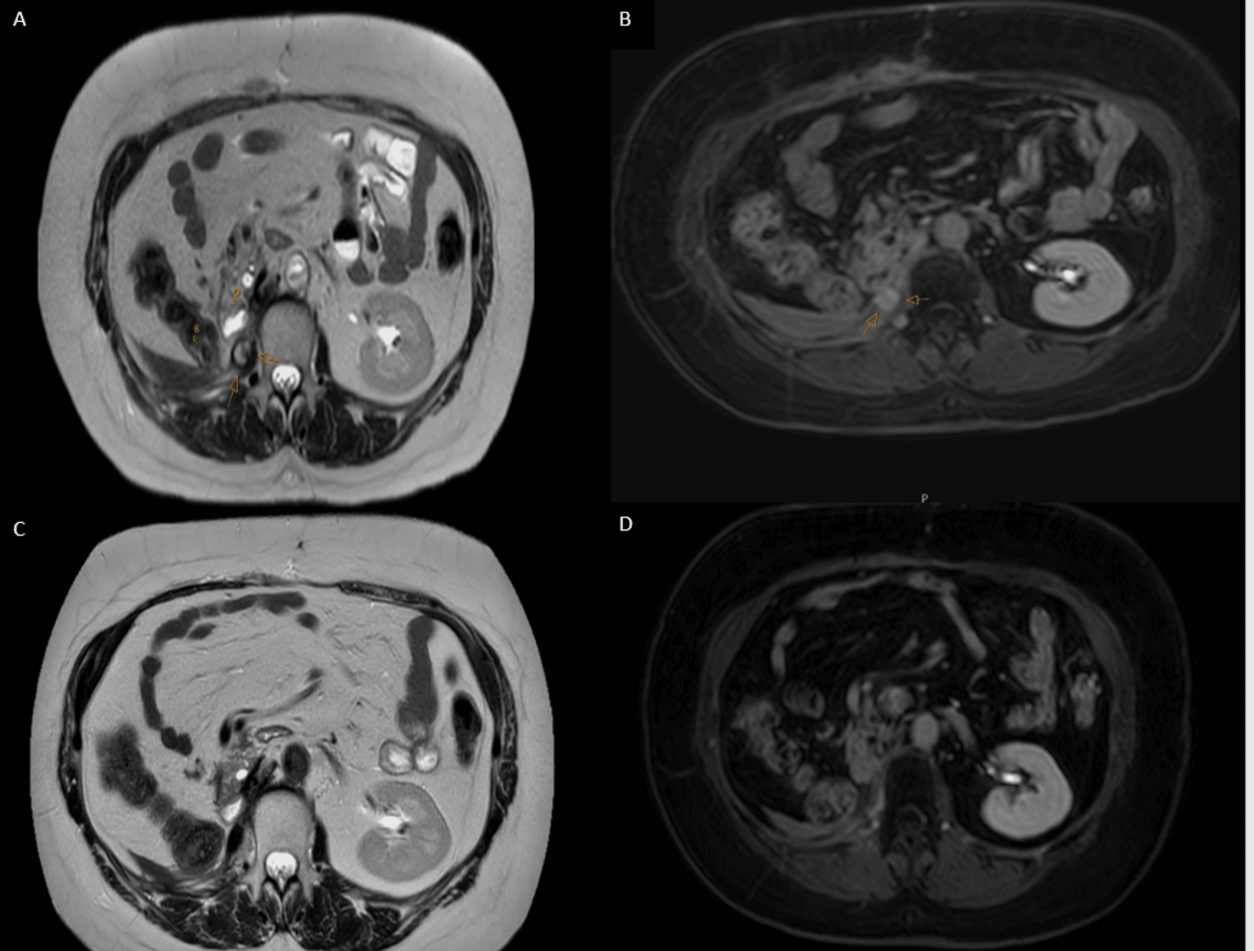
Radiographic response post-radiotherapy. The hyperintense signal seen in T2-weighted image (A) has resoluted (C). In addition, the prominent gadolinium enhancement (B) has significantly diminished (D).
